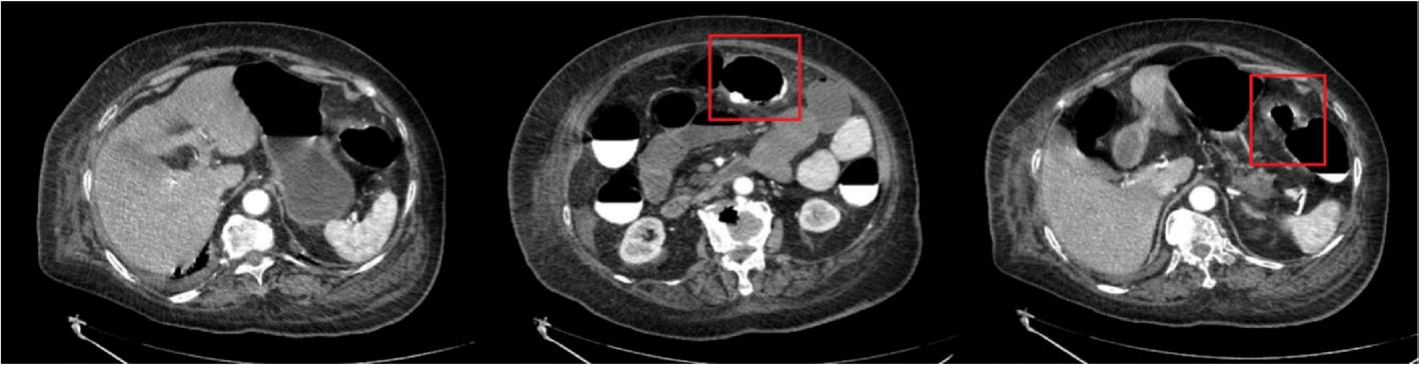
Contrast-Enhanced Abdominal CT (November 20, 2024)
